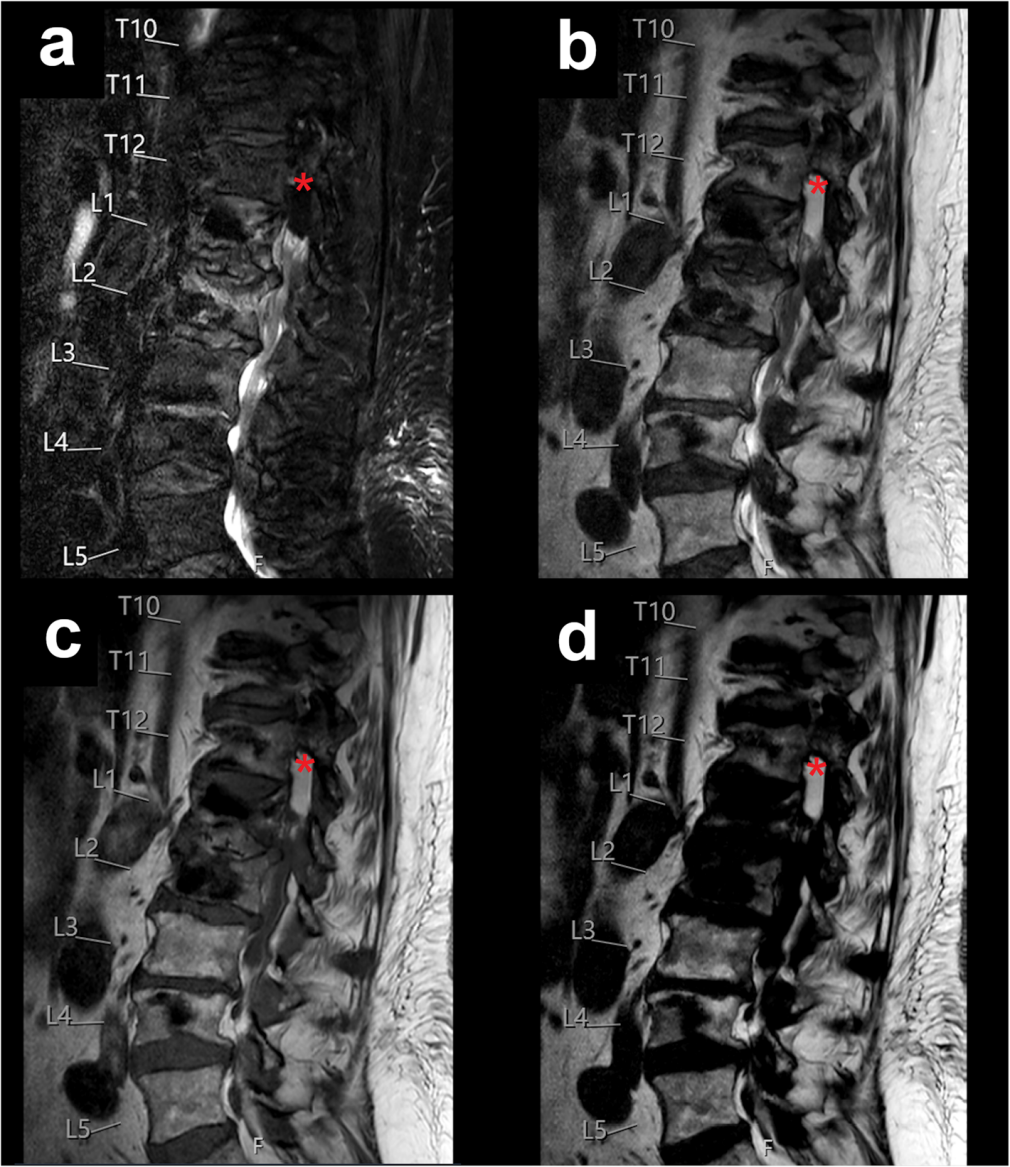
Postoperative lumbar haematoma. This figure shows the lumbar spine of an 80-year-old female patient using water-only images (a), in-phase images (b), and fat-only images (d) of the sagittal T2-weighted DIXON sequence, together with a sagittal T1-weighted sequence (c). The patient had undergone decompressive lumbar hemilaminectomy and presented with a small lateralised postoperative haematoma of the intradural extramedullary space between T12 and L1 (red asterisks)
